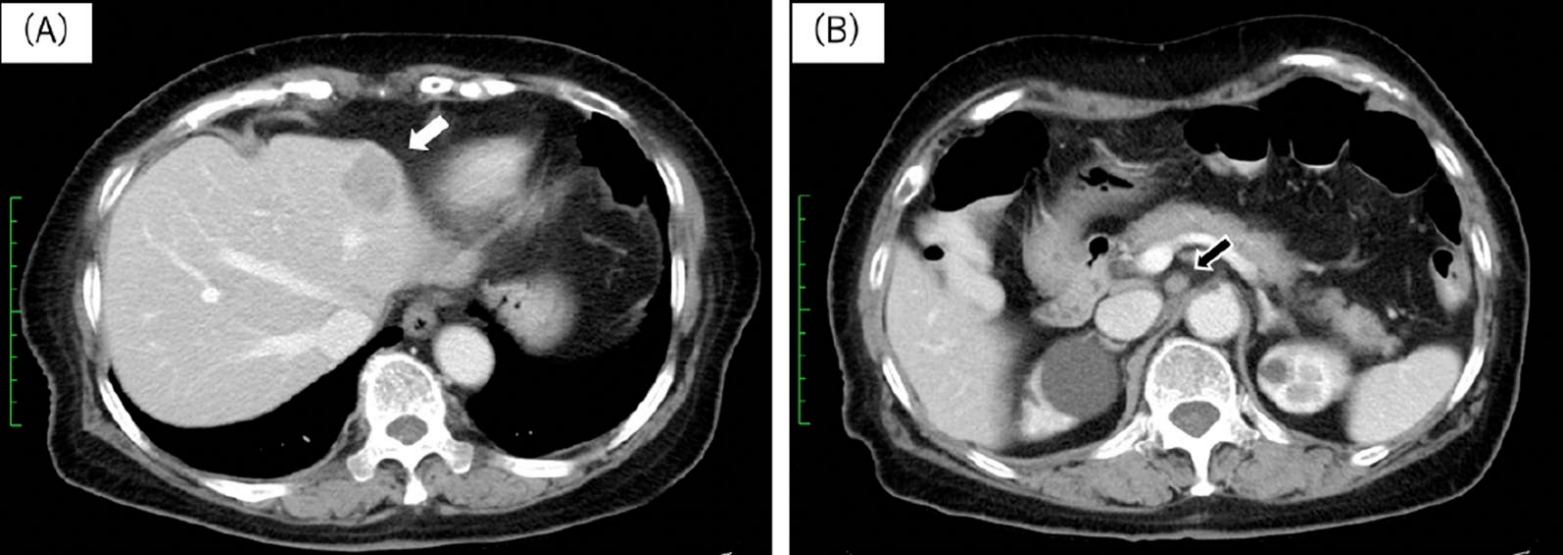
Preoperative CT findings. A CT scan showed a ring enhanced liver tumor (30 × 20 mm) in S3 liver lesion (white arrow). It was suspected a liver metastasis of sigmoid colon cancer. B CT scan showed an enlarged lymph node (10 × 10 mm) around abdominal aorta with contrast effect (black arrow). It was suspected a distant lymph node metastasis
